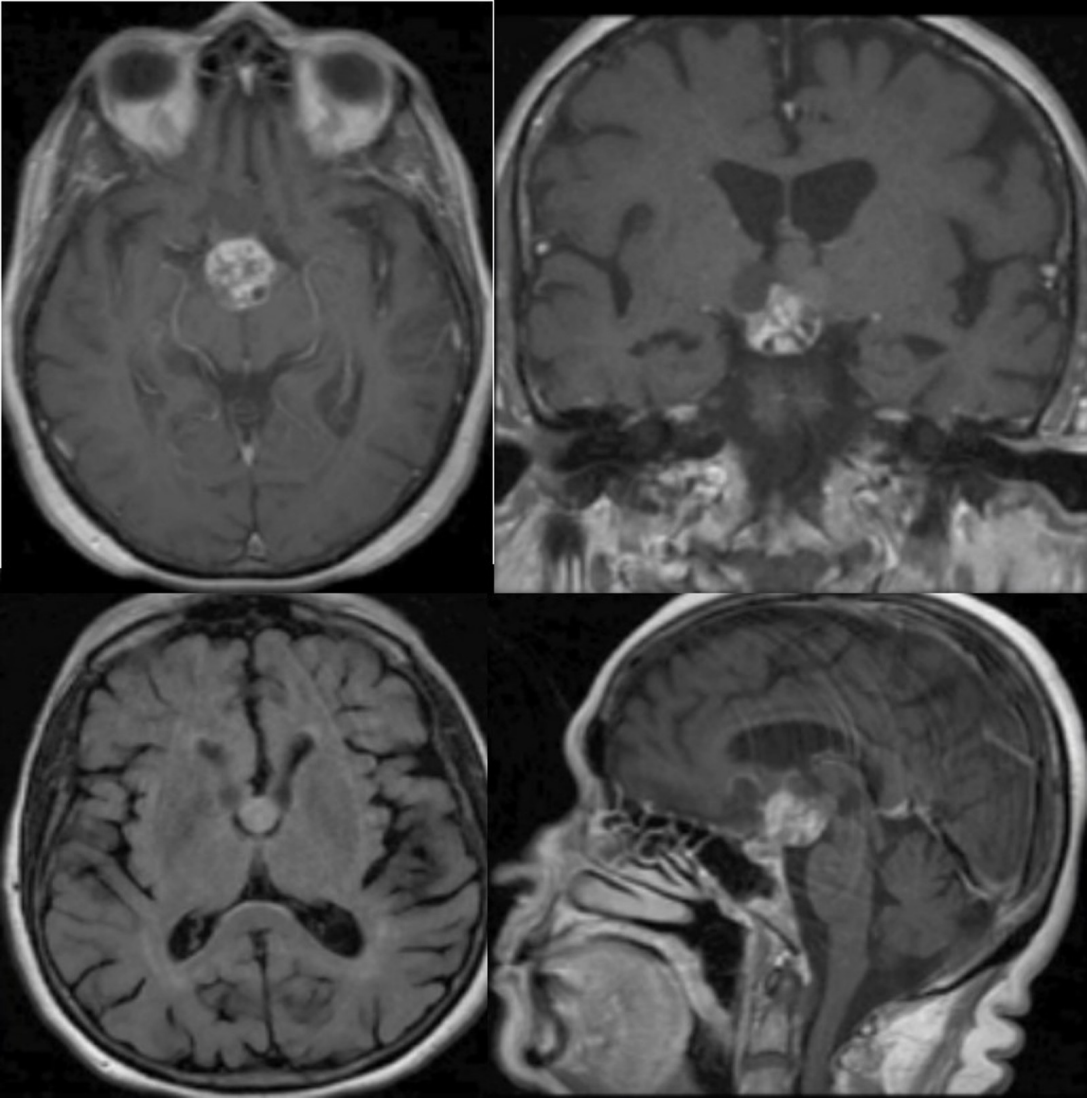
Preoperative MRI revealing a mass suspicious for craniopharyngioma Axial, coronal, and sagittal reconstructions of the patient's preoperative MRI. It depicts an enhancing lesion with a predominantly suprasellar component with third ventricular extension.
